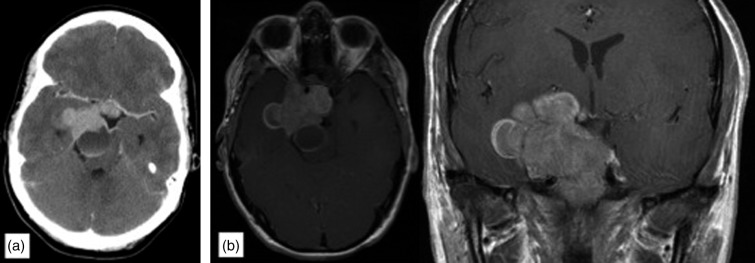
(a) Computed tomography of the head at presentation shows a large lobular/cystic mass invading the right cavernous sinus, displacing and compressing the midbrain. (b) Magnetic resonance image of the brain at presentation further characterises the mass (T1 sagittal and coronal).
